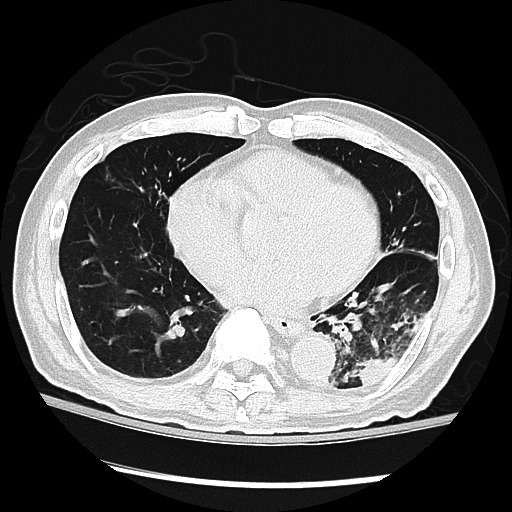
CT of *L. cardiaca* pneumonia in a 73-year-old man showing consolidative and ground-glass opacities in the left lower lobe and ground-glass appearance and bronchiectasis in the other lobes.
